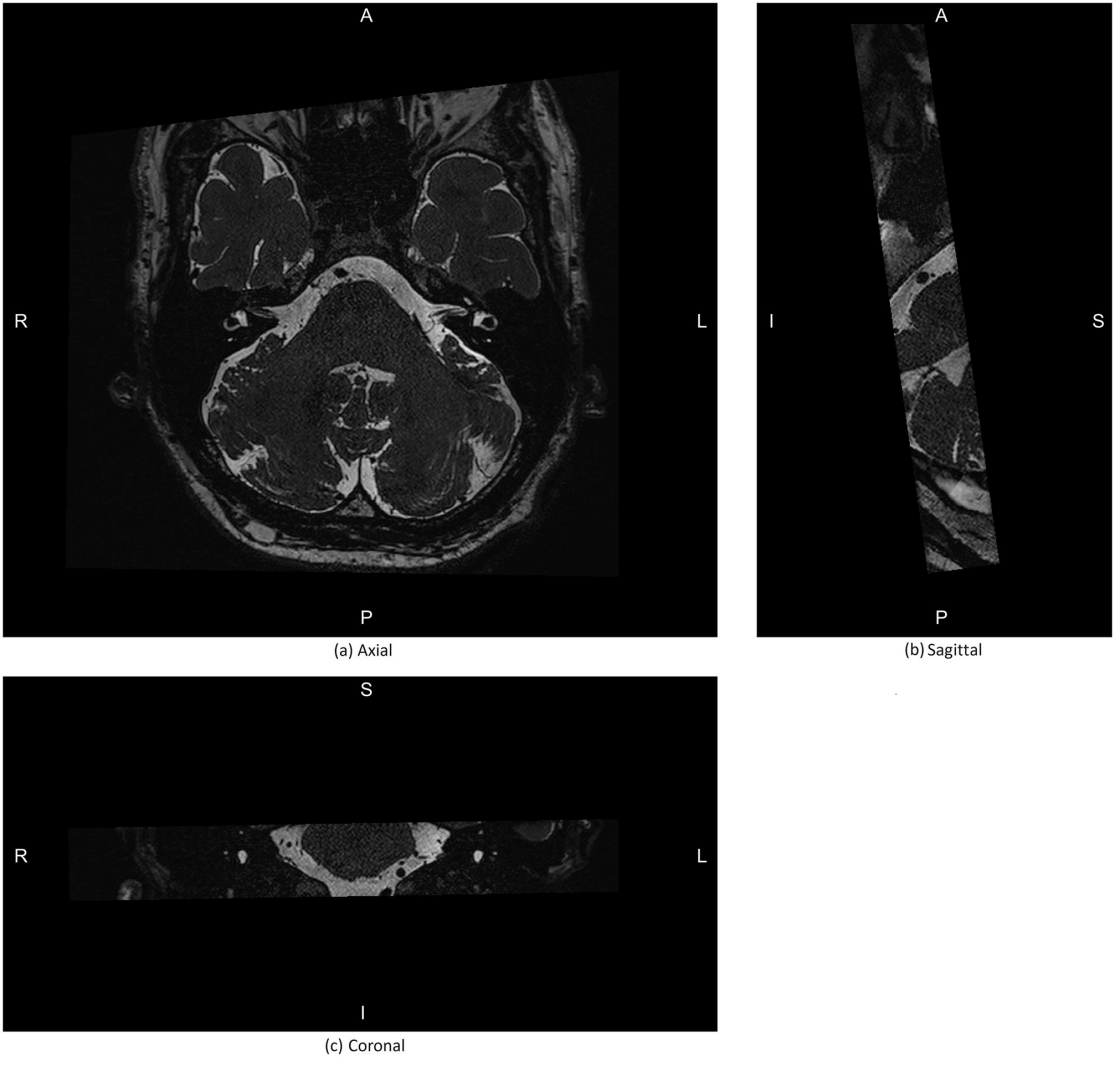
Mid slices of a MR image in the proposed coordinate system. A, P, S, I, R, and L indicate anterior, posterior, superior, inferior, right, and left directions respectively.
